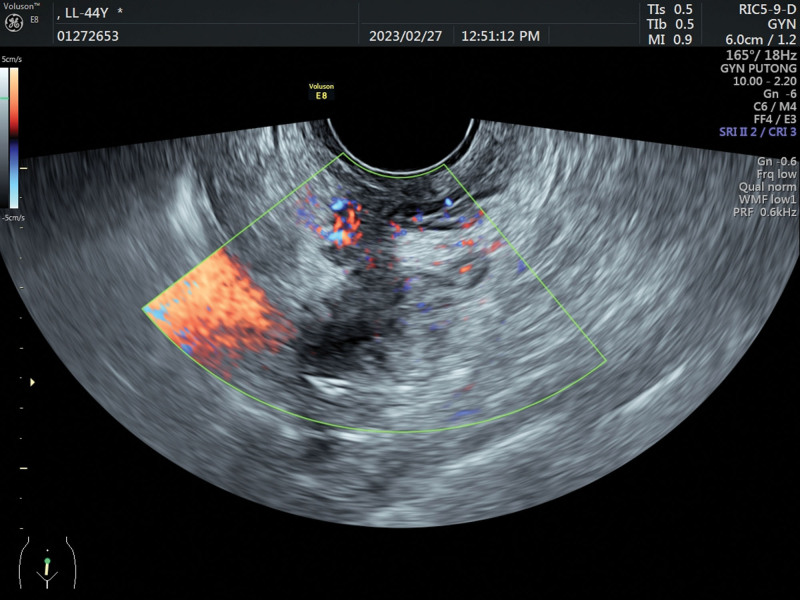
The lesion appears as a dendritic blood flow signal on color Doppler imaging.
